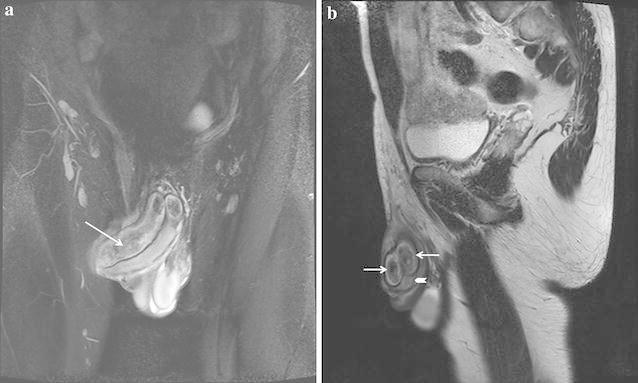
Magnetic resonance imaging of the erected penis. MRI (Heavily T2-weighted image) shows both sides of corpus cavernosum (*arrow*) increased significant thickly, as well as the corpus spongiosum (*arrowhead*). a Coronal view, b sagittal view
